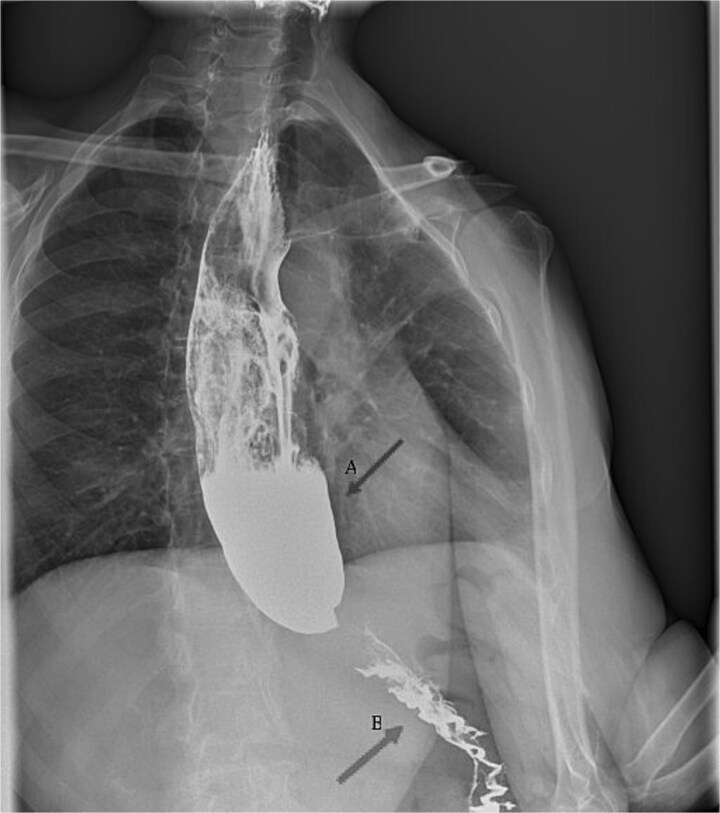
Barium swallow X-ray (January 2024) showing dilatation of the oesophagus (arrow A) and a tapered narrowing at the oesophagogastric junction (‘bird-beak sign’) (arrow B).
